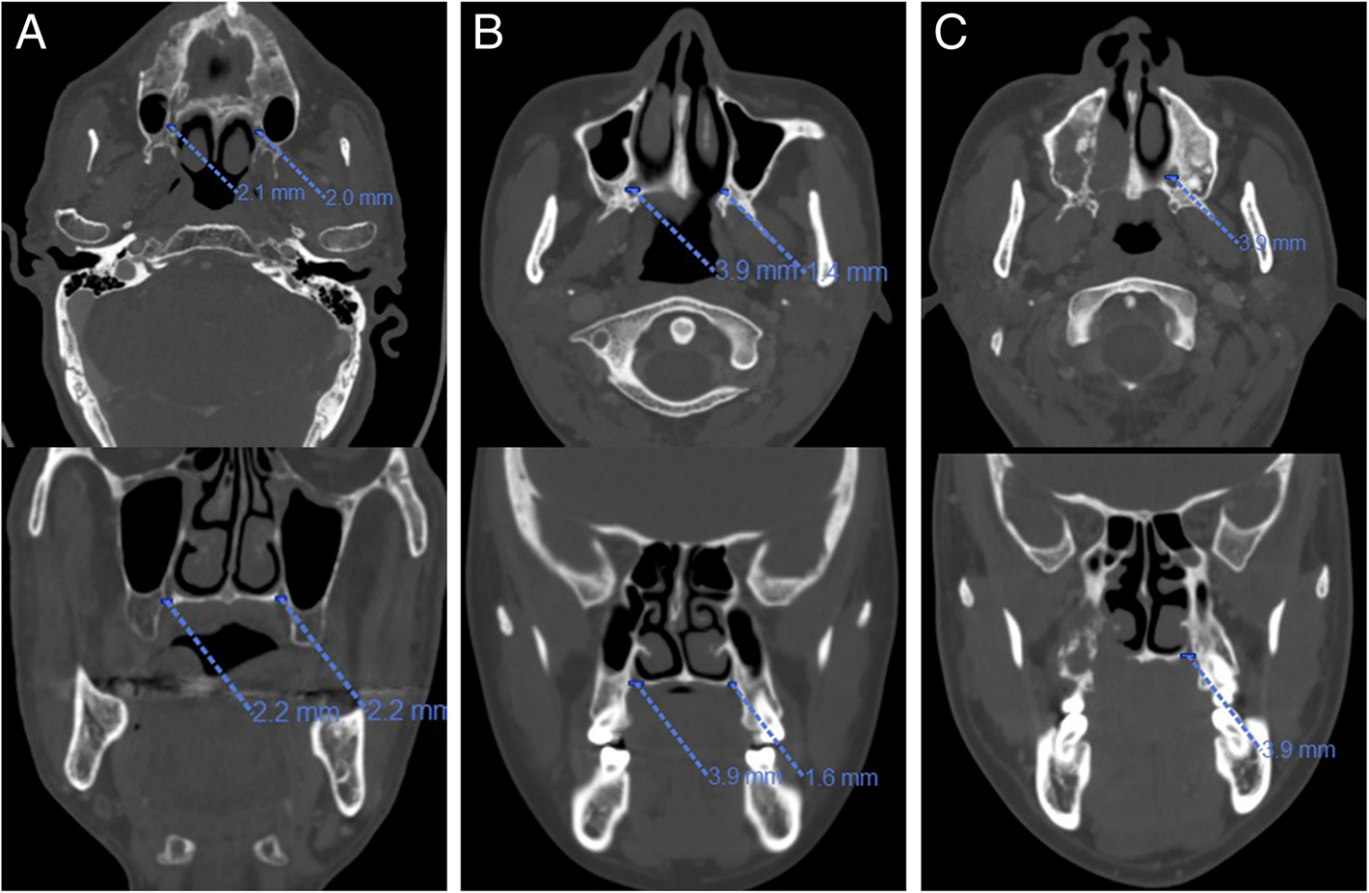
Computed tomographic images of cases with or without GPF enlargement. The diameters of the left and right GPFs were measured in bone window (upper layer: horizontal level; lower layer: coronal reconstruction). (a) No.35 patient was considered without GPF enlargement (GPF diameter on the tumor side was 0.2 mm larger than that on the normal side). (b) No.13 patient was considered with GPF enlargement (GPF diameter on the tumor side was more than 0.3 mm larger than that on the normal side). (c) NO. 71 patient was considered as GPF enlargement (complete destruction of GPF on the tumor side). GPF = greater palatine foramen
